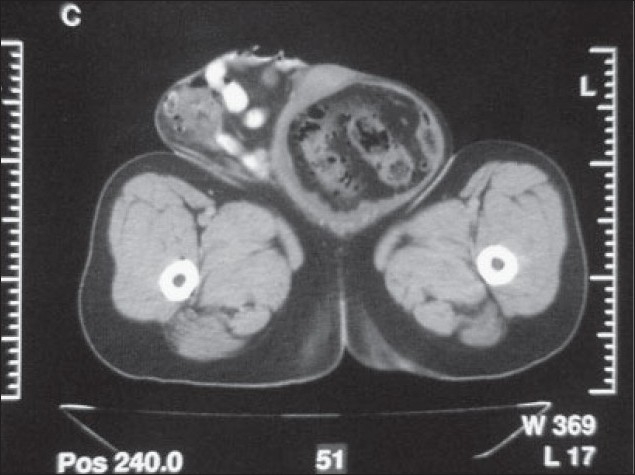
Intestinal segments at the level of femur on computed tomography.
